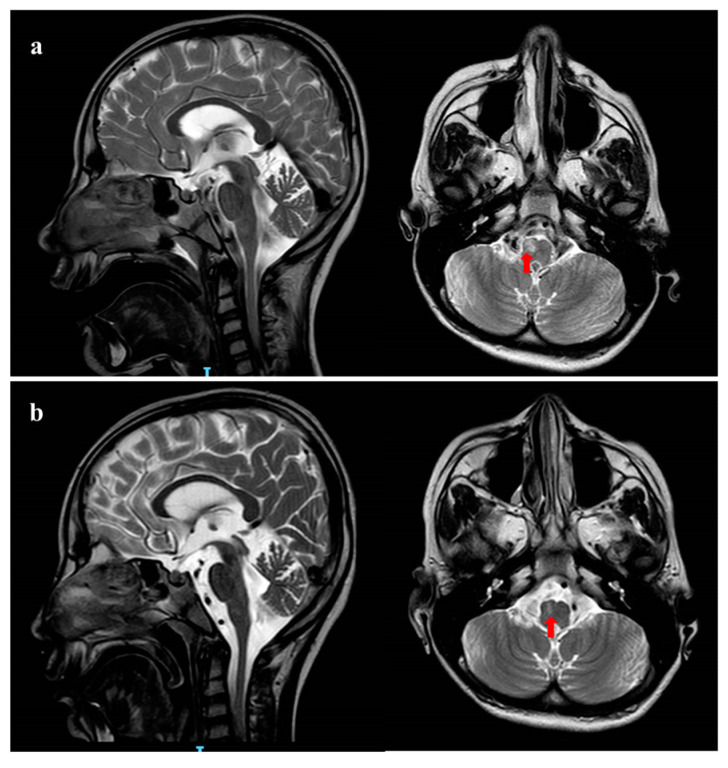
Brain MRI pattern. (a) Brain MRI performed in the acute phase at 10 years of age. Coronal and Sagittal T2 weighted images shows global cerebral and cerebellar atrophy. Red arrow shows hyperintensity of the right bulbar pyramid; (b) brain MRI performed 20 days later shows reduction in the right corresponding bulbar pyramid lesion.
